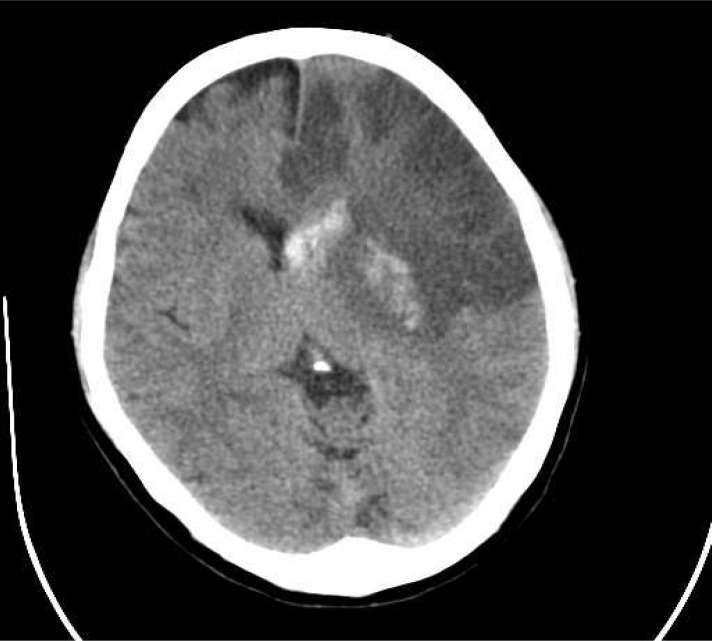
CT scan 48 h after tissue plasminogen activator administration
